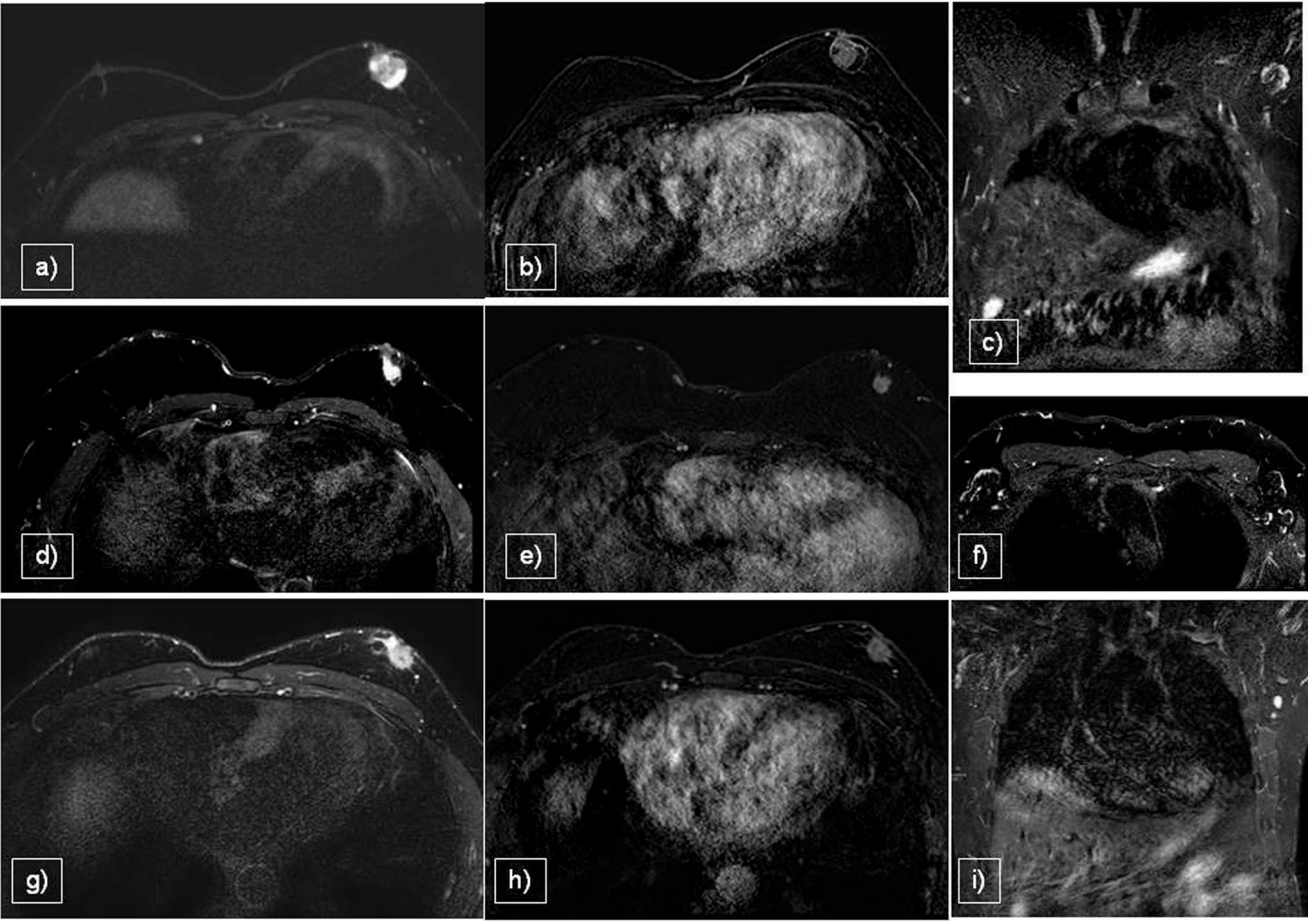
Papillary carcinoma a,b,c, (IDC) G2 d,e,f, and IDC G3 g,h,i presented in three sequences: STIR, CE T1WI and STIR for axillary evaluation. MRI demonstrated lesions with irregular margins and enhancement, nipple/skin infiltration (b,h), and node involvement seen only in IDC G3 (i)
